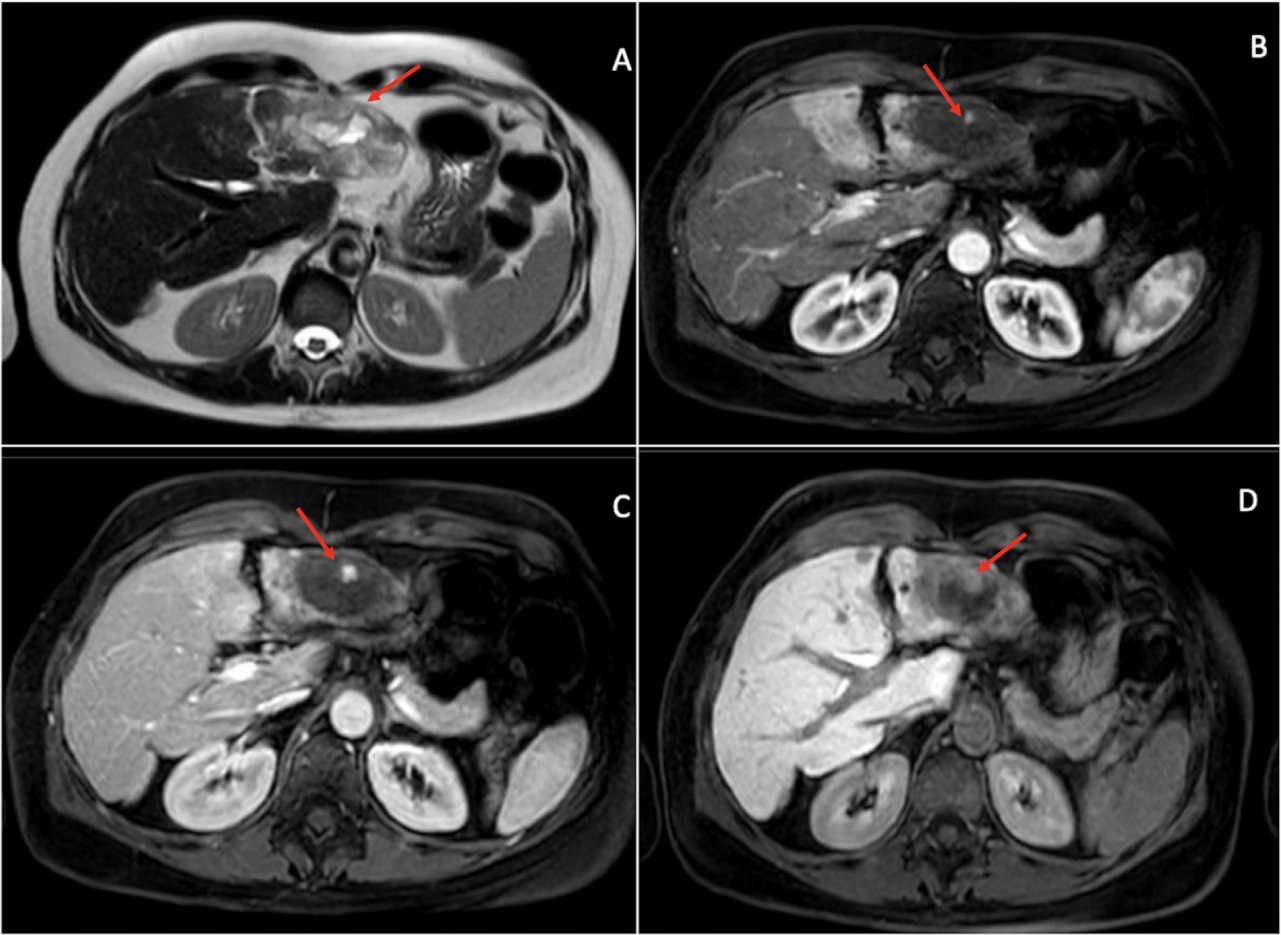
Magnetic resonance imaging with hepatospecific contrast agent. At the level of the II/III hepatic segment a non-homogeneously hyperintense signal alteration was documented in the T2-weighted sequences (A). Central vascular pooling is evident in the arterial (B) and venous (C) phases with irregular signal and centrifugal pattern. Minimal peripheral impregnation of the contrast agent was observed in the hepatobiliary phase (D).
